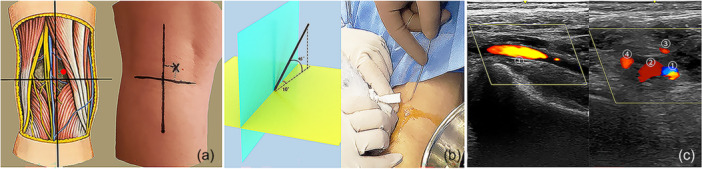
Ultrasound‐assisted positioning for insertion of K‐wire. (a) Entry point of wire was 1 cm each away from vertical and horizontal axes in the medial‐upper quadrants. (b) K‐wire was placed at angles of 10° in reference to the sagittal plane (blue) and 45° in reference to the coronal plane (yellow). (c) Neurovascular structures were identified in the sagittal and transverse plane under B‐ultrasound monitoring: (1) popliteal artery, (2) popliteal vein, (3) medial inferior genicular vein and (4) lateral inferior genicular vein. K‐wire, Kirschner wire.
